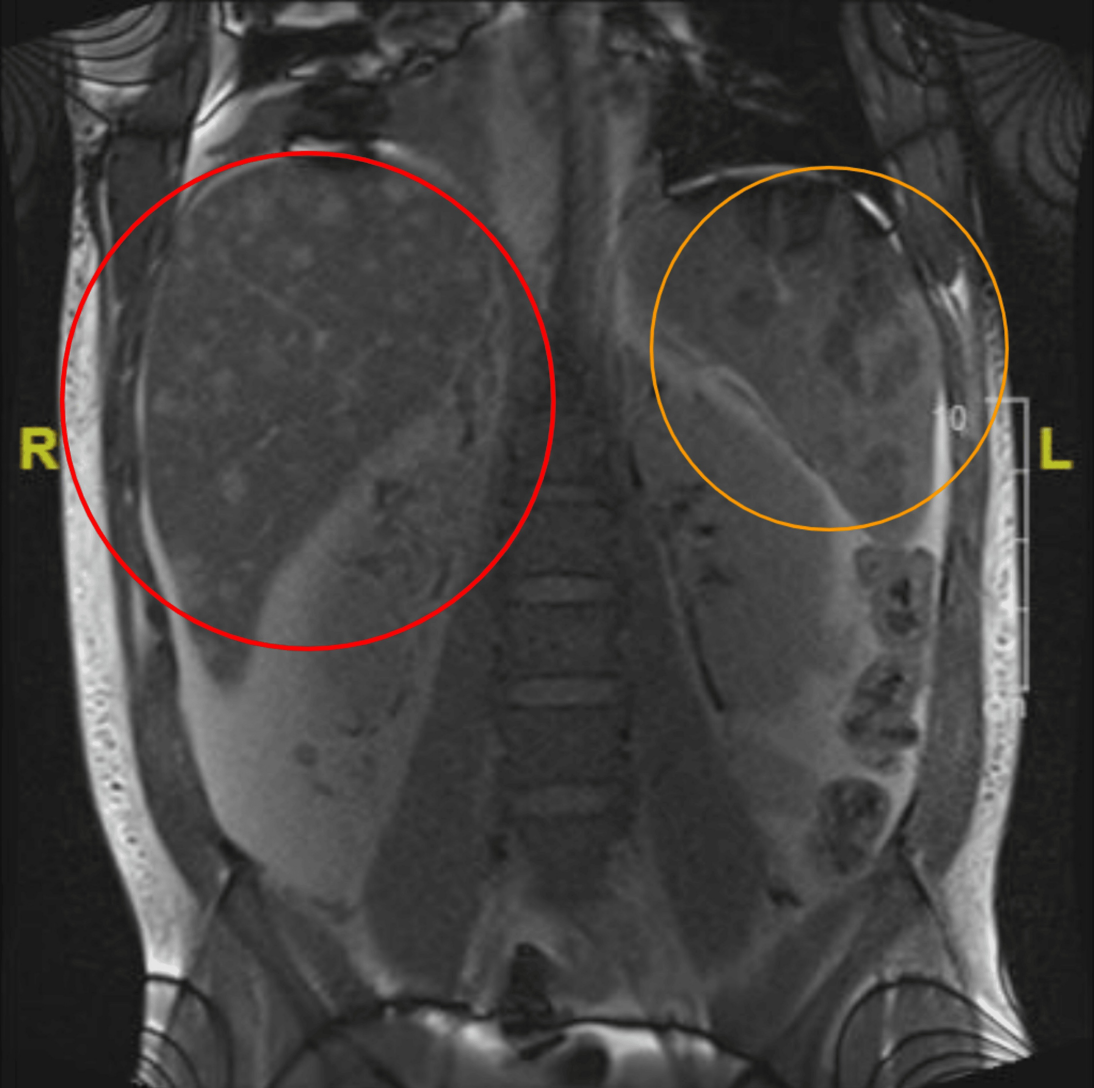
MRI without contrast (coronal view) The image displays hepatosplenomegaly with multiple nodular lesions in both liver (red circle) and spleen (orange circle) MRI: magnetic resonance imaging
